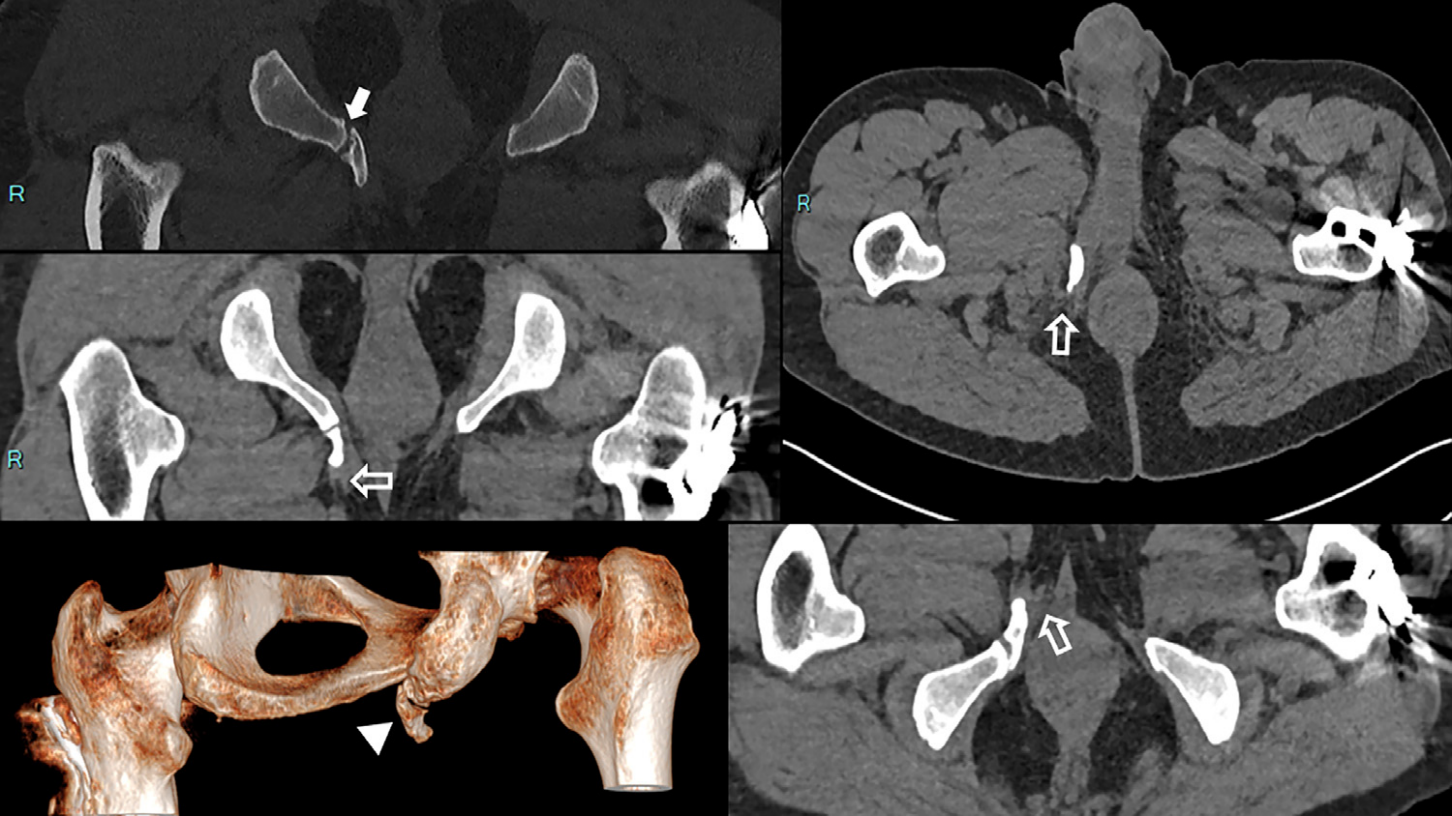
Pelvic CT scan. The pelvic digit extends anteriorly and demonstrates a pseudoarthrotic articulation with the right ischiopubic branch (white arrow). The 3D reconstruction (white arrowhead) highlights its anteroinferior orientation. Note the soft tissue infiltration around the distal end of the digit, particularly in the subcutaneous fat, likely due to chronic mechanical irritation in the sitting position (white underlined arrow).
